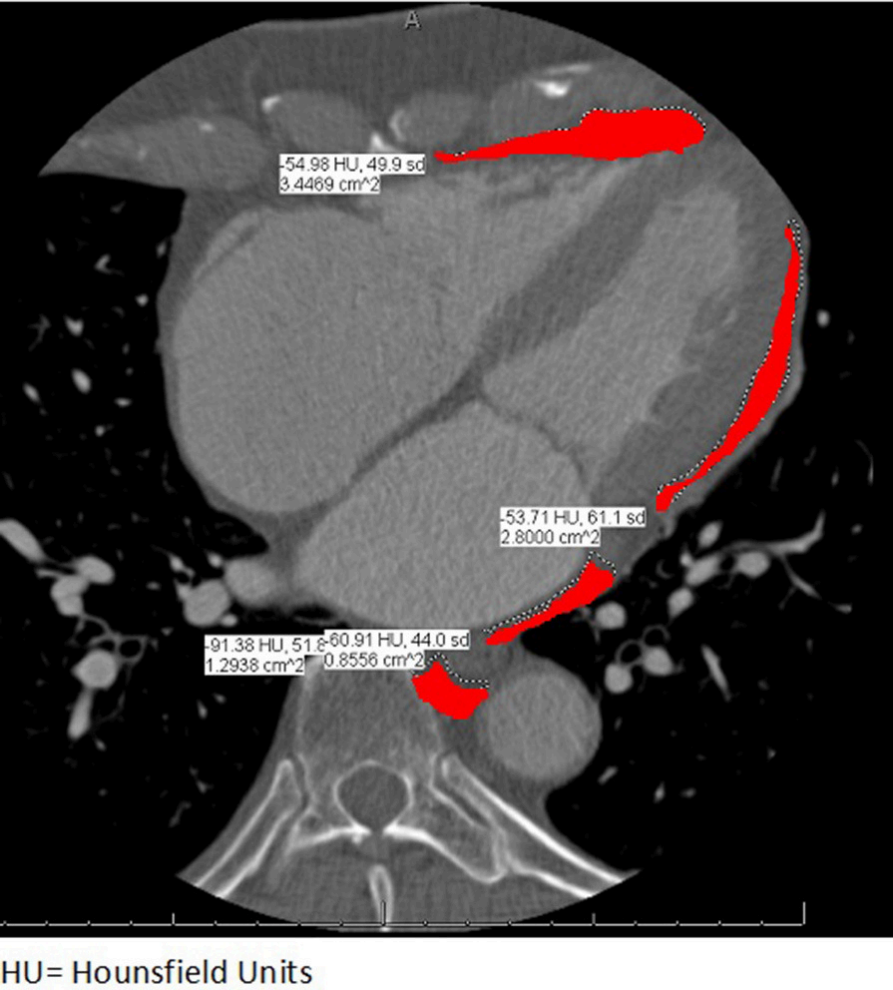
Example of a study participant showing EAT measurement in a single CT image using a 4-chamber equivalent view. Areas in red depict traced EAT with their respective areas and Hounsfield Units.
